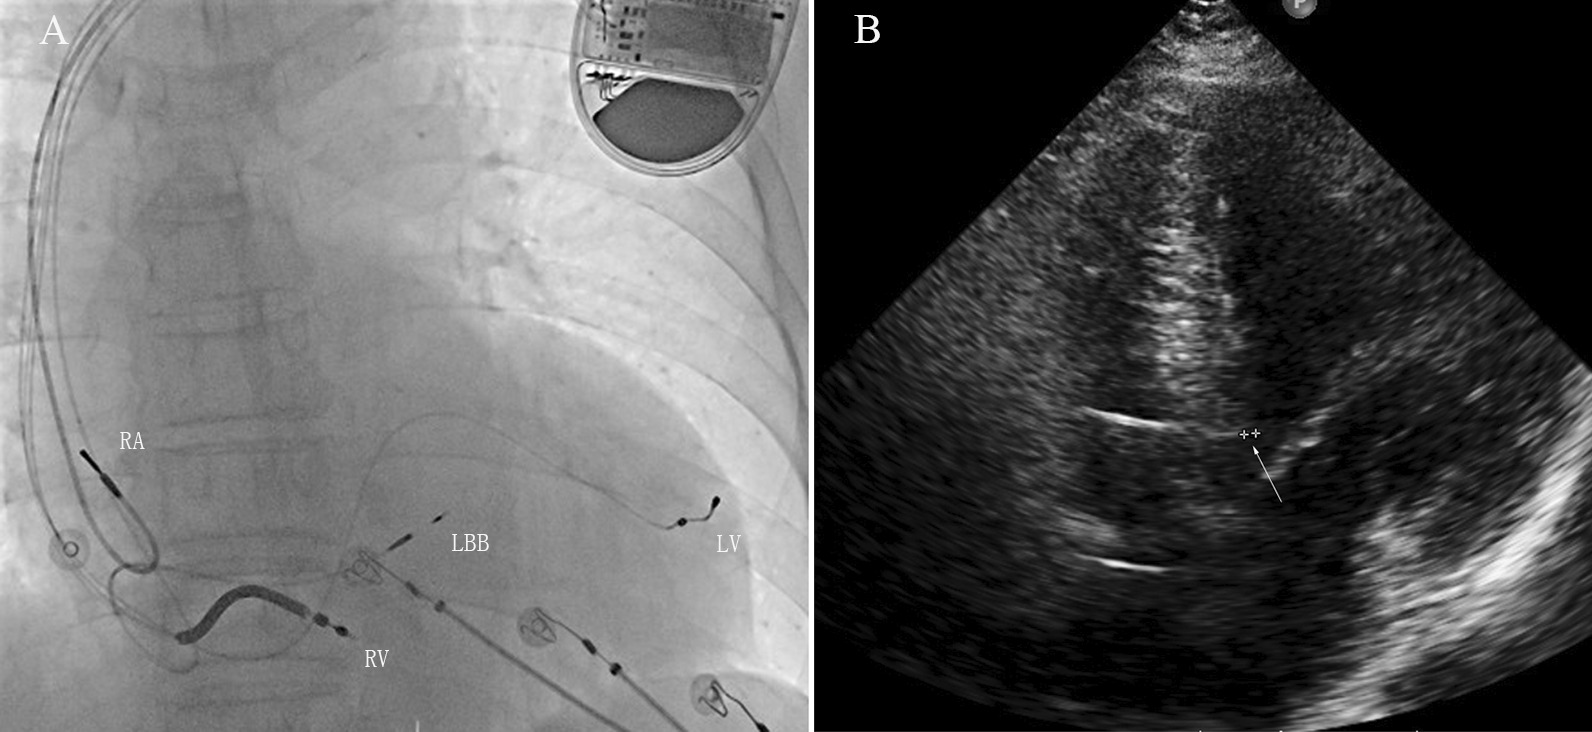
Fluoroscopic image and echo image of an LOT-aCRTD in a patient with ischaemic cardiomyopathy and a normal PR interval. a Fluoroscopic image in the RAO 30° projections. This image shows the final LBB lead position in the interventricular septum. *RA* right atrial lead, *LV* coronary sinus lead, *LBB* left bundle branch lead, *RV* right ventricular defibrillator lead. b Transthoracic echocardiogram image. The apical four-chamber view demonstrates the depth of the LBB lead in the interventricular septum (arrow)
